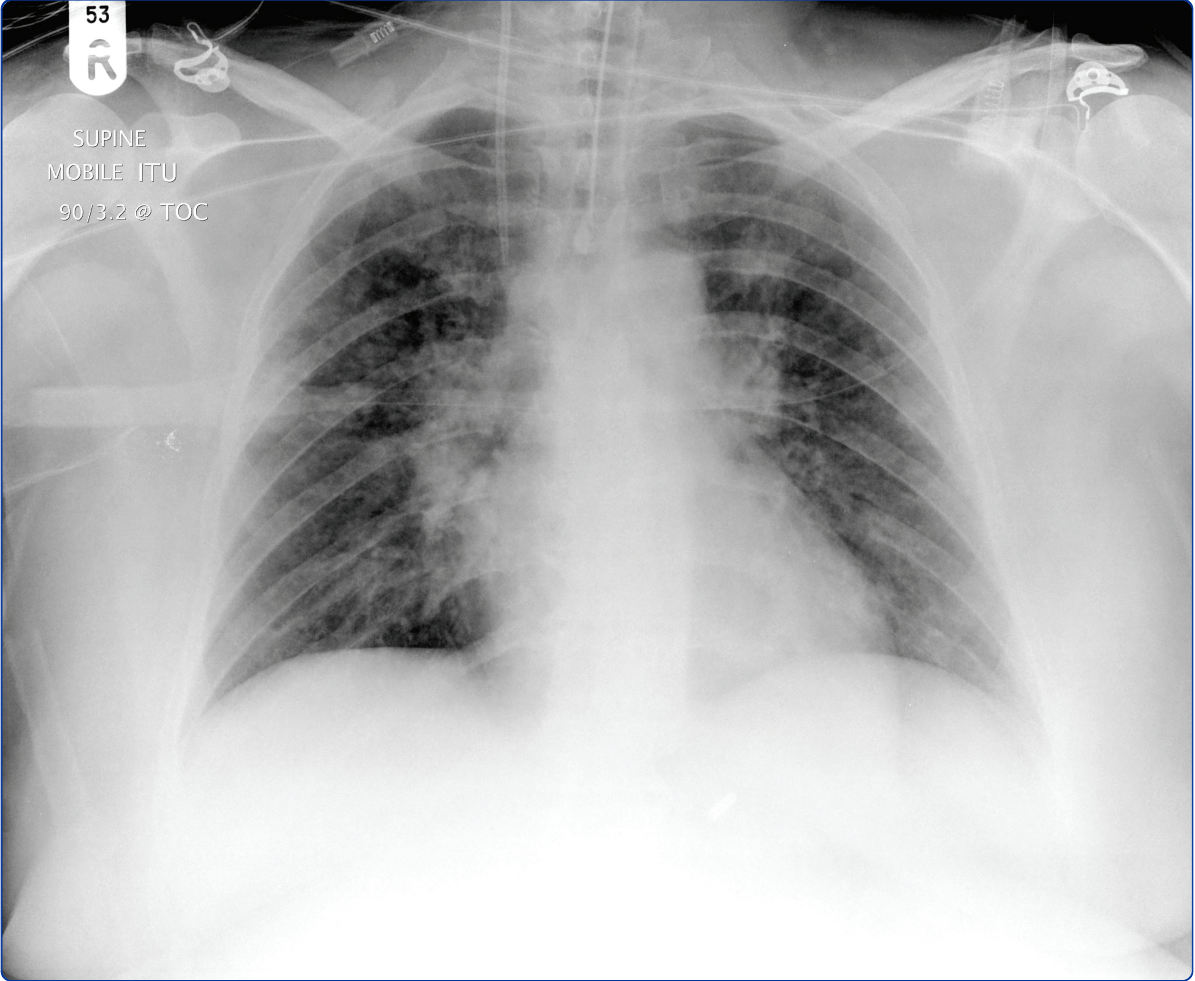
The patient's repeat chest radiograph showing widespread bilateral interstitial infiltrates.
